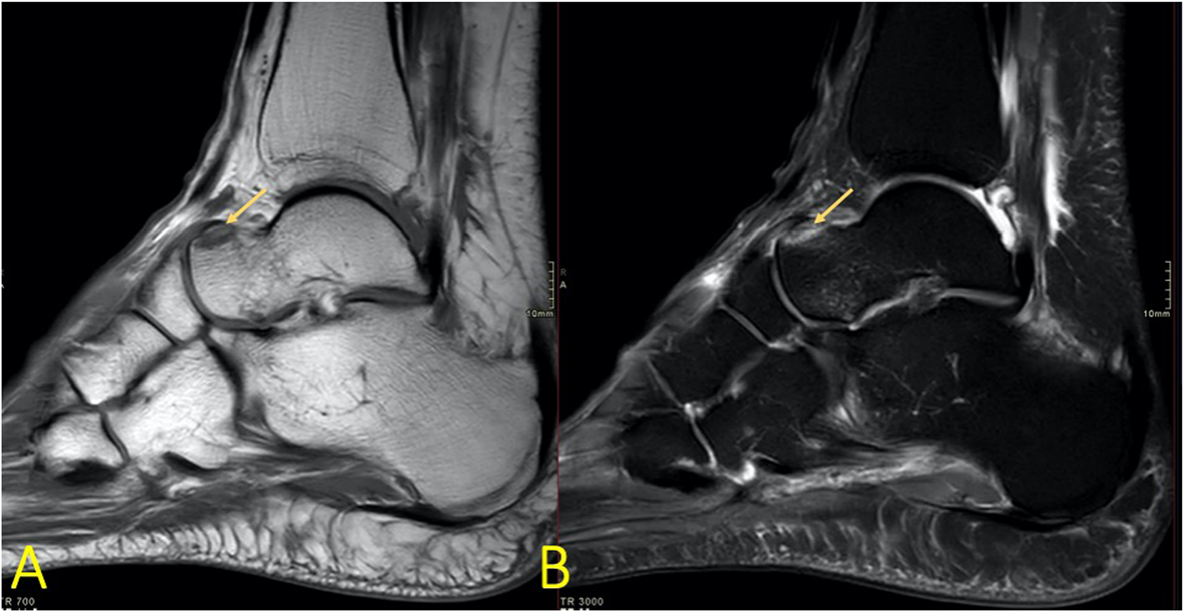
A 19-year-old soccer player sustained an ankle sprain during a soccer match with suspicion of rupture of the anterior tibiofibular ligament. MRI showed avulsion (arrow) of the talar attachment of the dorsal talonavicular ligament
